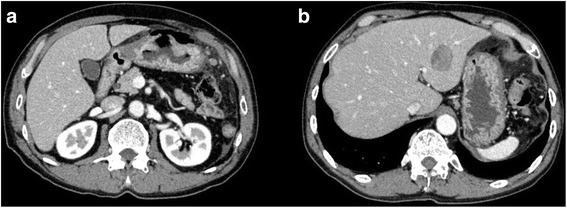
Imaging findings. Abdominal contrast-enhanced computed tomography revealed disruption and thickening of the anterior wall of the gastric antrum with free air (a) and a low enhanced lesion (30 mm in diameter) on the lateral segment of the liver (b)
